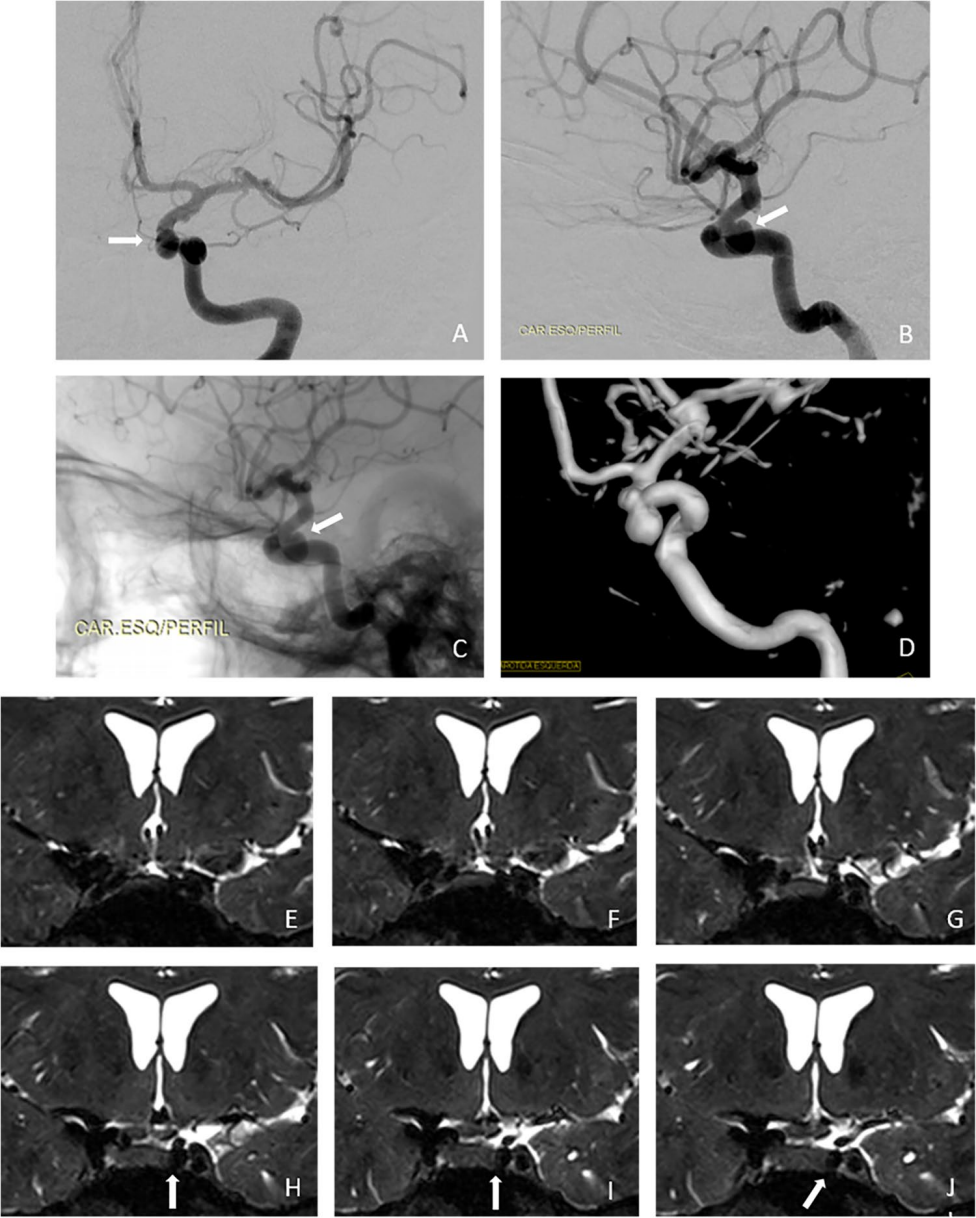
Cerebral digital subtraction angiography (DSA) of aneurysm #5 that caused intertechnique disagreement (see Table 1) showing anteroposterior (panel A) and profile (panel B) views. Note the medial projection of the aneurysm (white arrows). The relationship between the aneurysm and bony structures are shown (panels C and D). Coronal T2-weighted magnetic resonance imaging (MRI) scans with a 3D fast spin-echo sequence showing anterior to posterior views of the anatomoradiological markers of the paraclinoid region and the intracavernous (MRI)/transitional (M) aneurysm #5 (panels G–J; aneurysm — white arrows)
